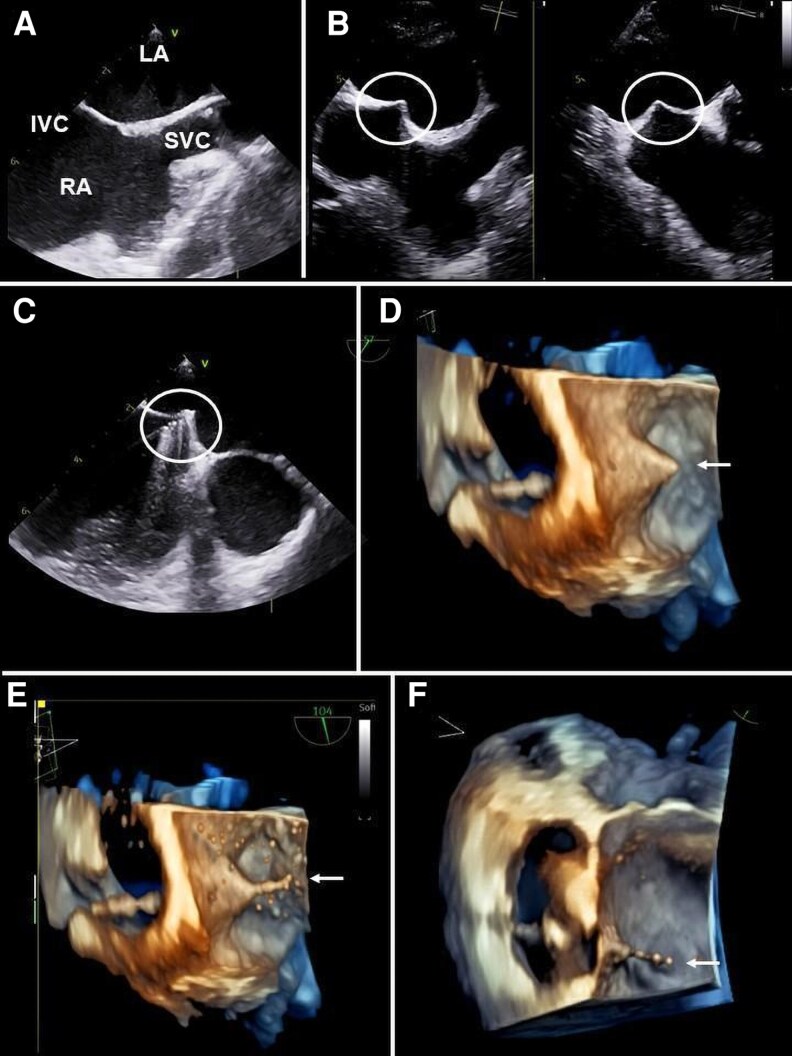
TOE plays a pivotal role in ensuring safe and accurate transseptal access. (*A*) Standard ME bicaval view showing the catheter-guide advancing from the IVC towards the fossa ovalis and the needle approaching the septum; proper identification of the fossa ovalis is crucial. (*B*) Biplane imaging enables accurate positioning and orientation of the catheter tip. (*C*) Needle pressure on the fossa ovalis procedures the typically ‘tenting’ sign (white circle). (*D*) 3D zoom en-face view of the fossa ovalis from the RA facilitates manipulation of the dilator; the white arrow indicates IAS tenting produced by the transseptal sheath. (*E* and *F*) Once adequate tenting is obtained, 3D zoom confirms correct and stable passage of the electrocautery system and wire across the fossa ovalis (white arrows). IAS: interatrial septum; IVC: inferior vena cava; LA: left atrium; ME: mid-oesophageal; RA: right atrium; SVC: superior vena cava; TOE: transoesophageal echocardiography.
